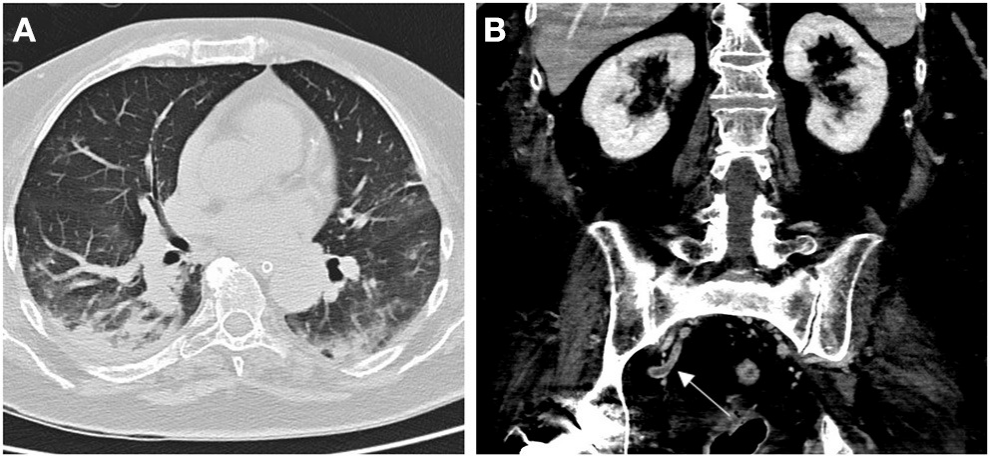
Chest CT scan showing typical COVID-19 associated bilateral pneumonia ad admission (A). Contrast-enhanced abdominal CT scan showing right internal vein thrombosis (arrow; B).
